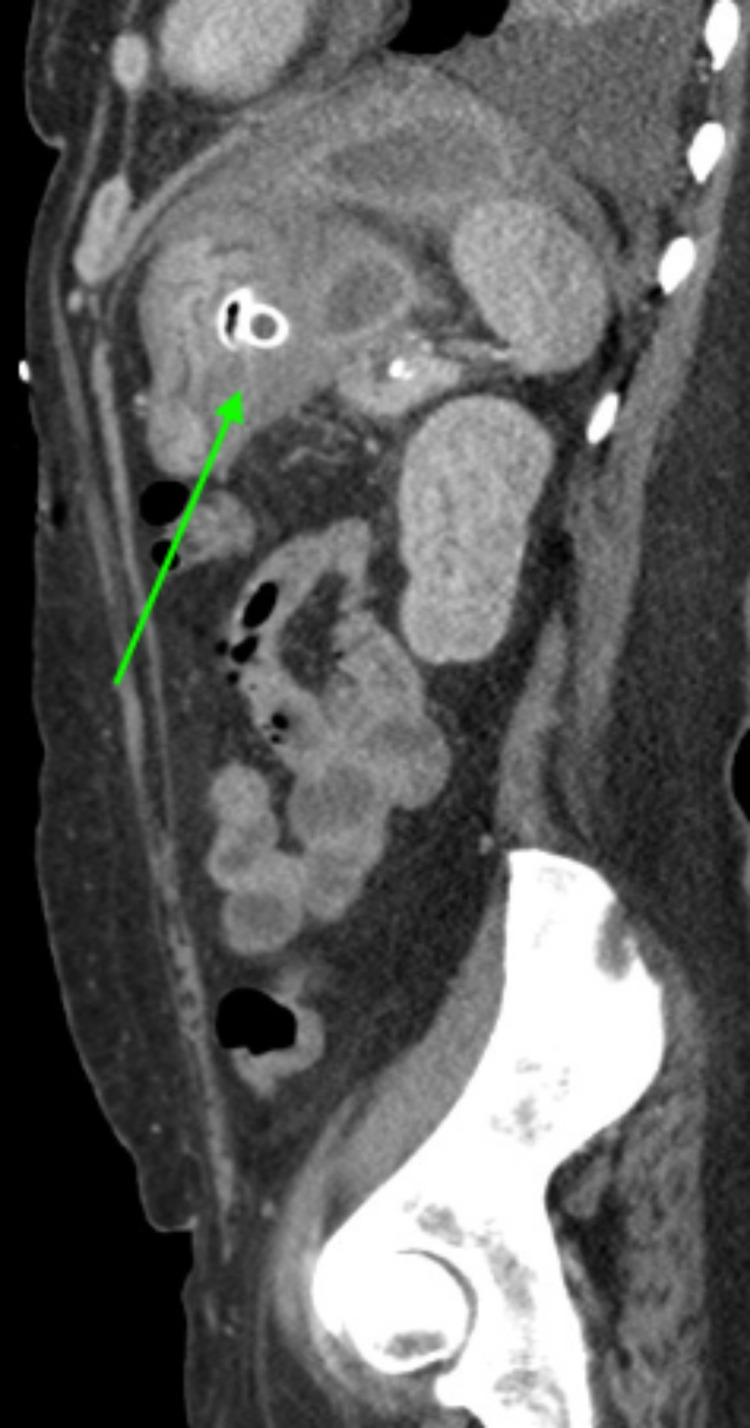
Sagittal view CT of the abdomen and pelvis with intravenous contrast post cystogastrostomy stent (arrow) placement demonstrating decompression of the giant pseudocyst. Residual gas and fluid are present in the collection. CT - computed tomography
